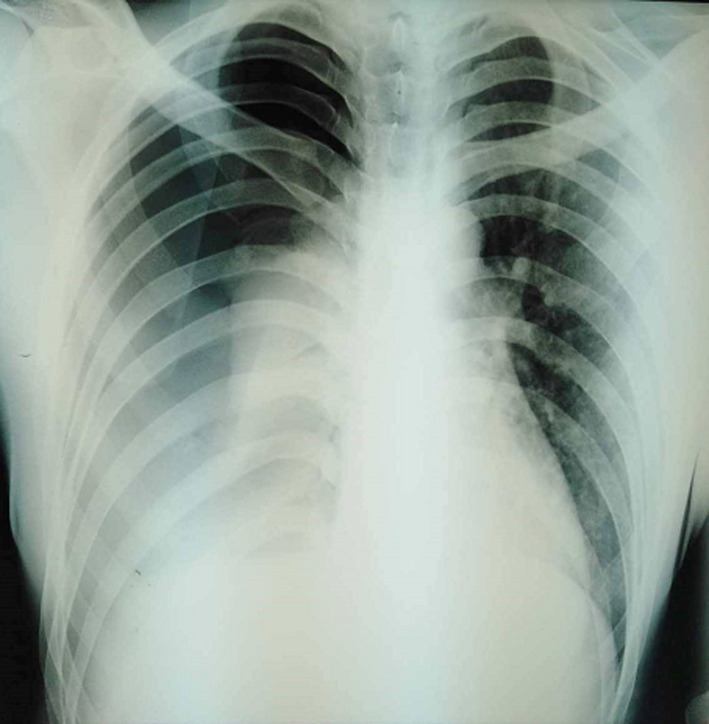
chest X-ray showing large, complete and compressive right-sided pneumothorax associated to pleural effusion
